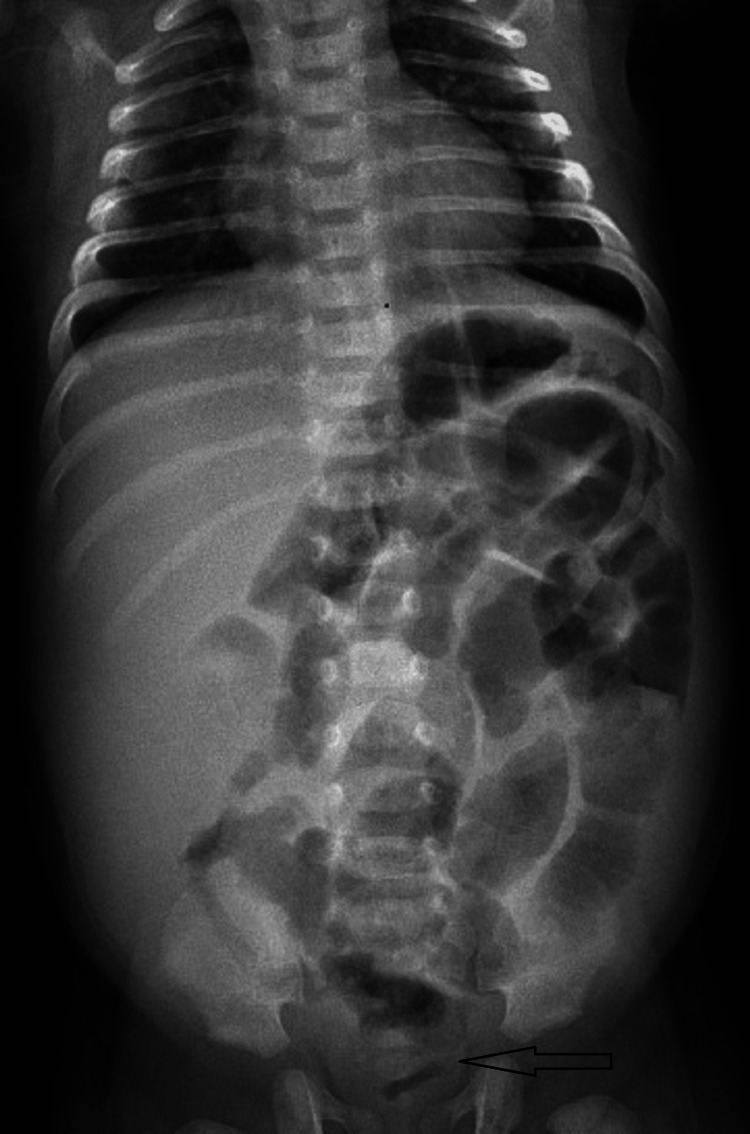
X-ray of the abdomen showing a gas shadow reaching the rectum and no pneumoperitoneum.
